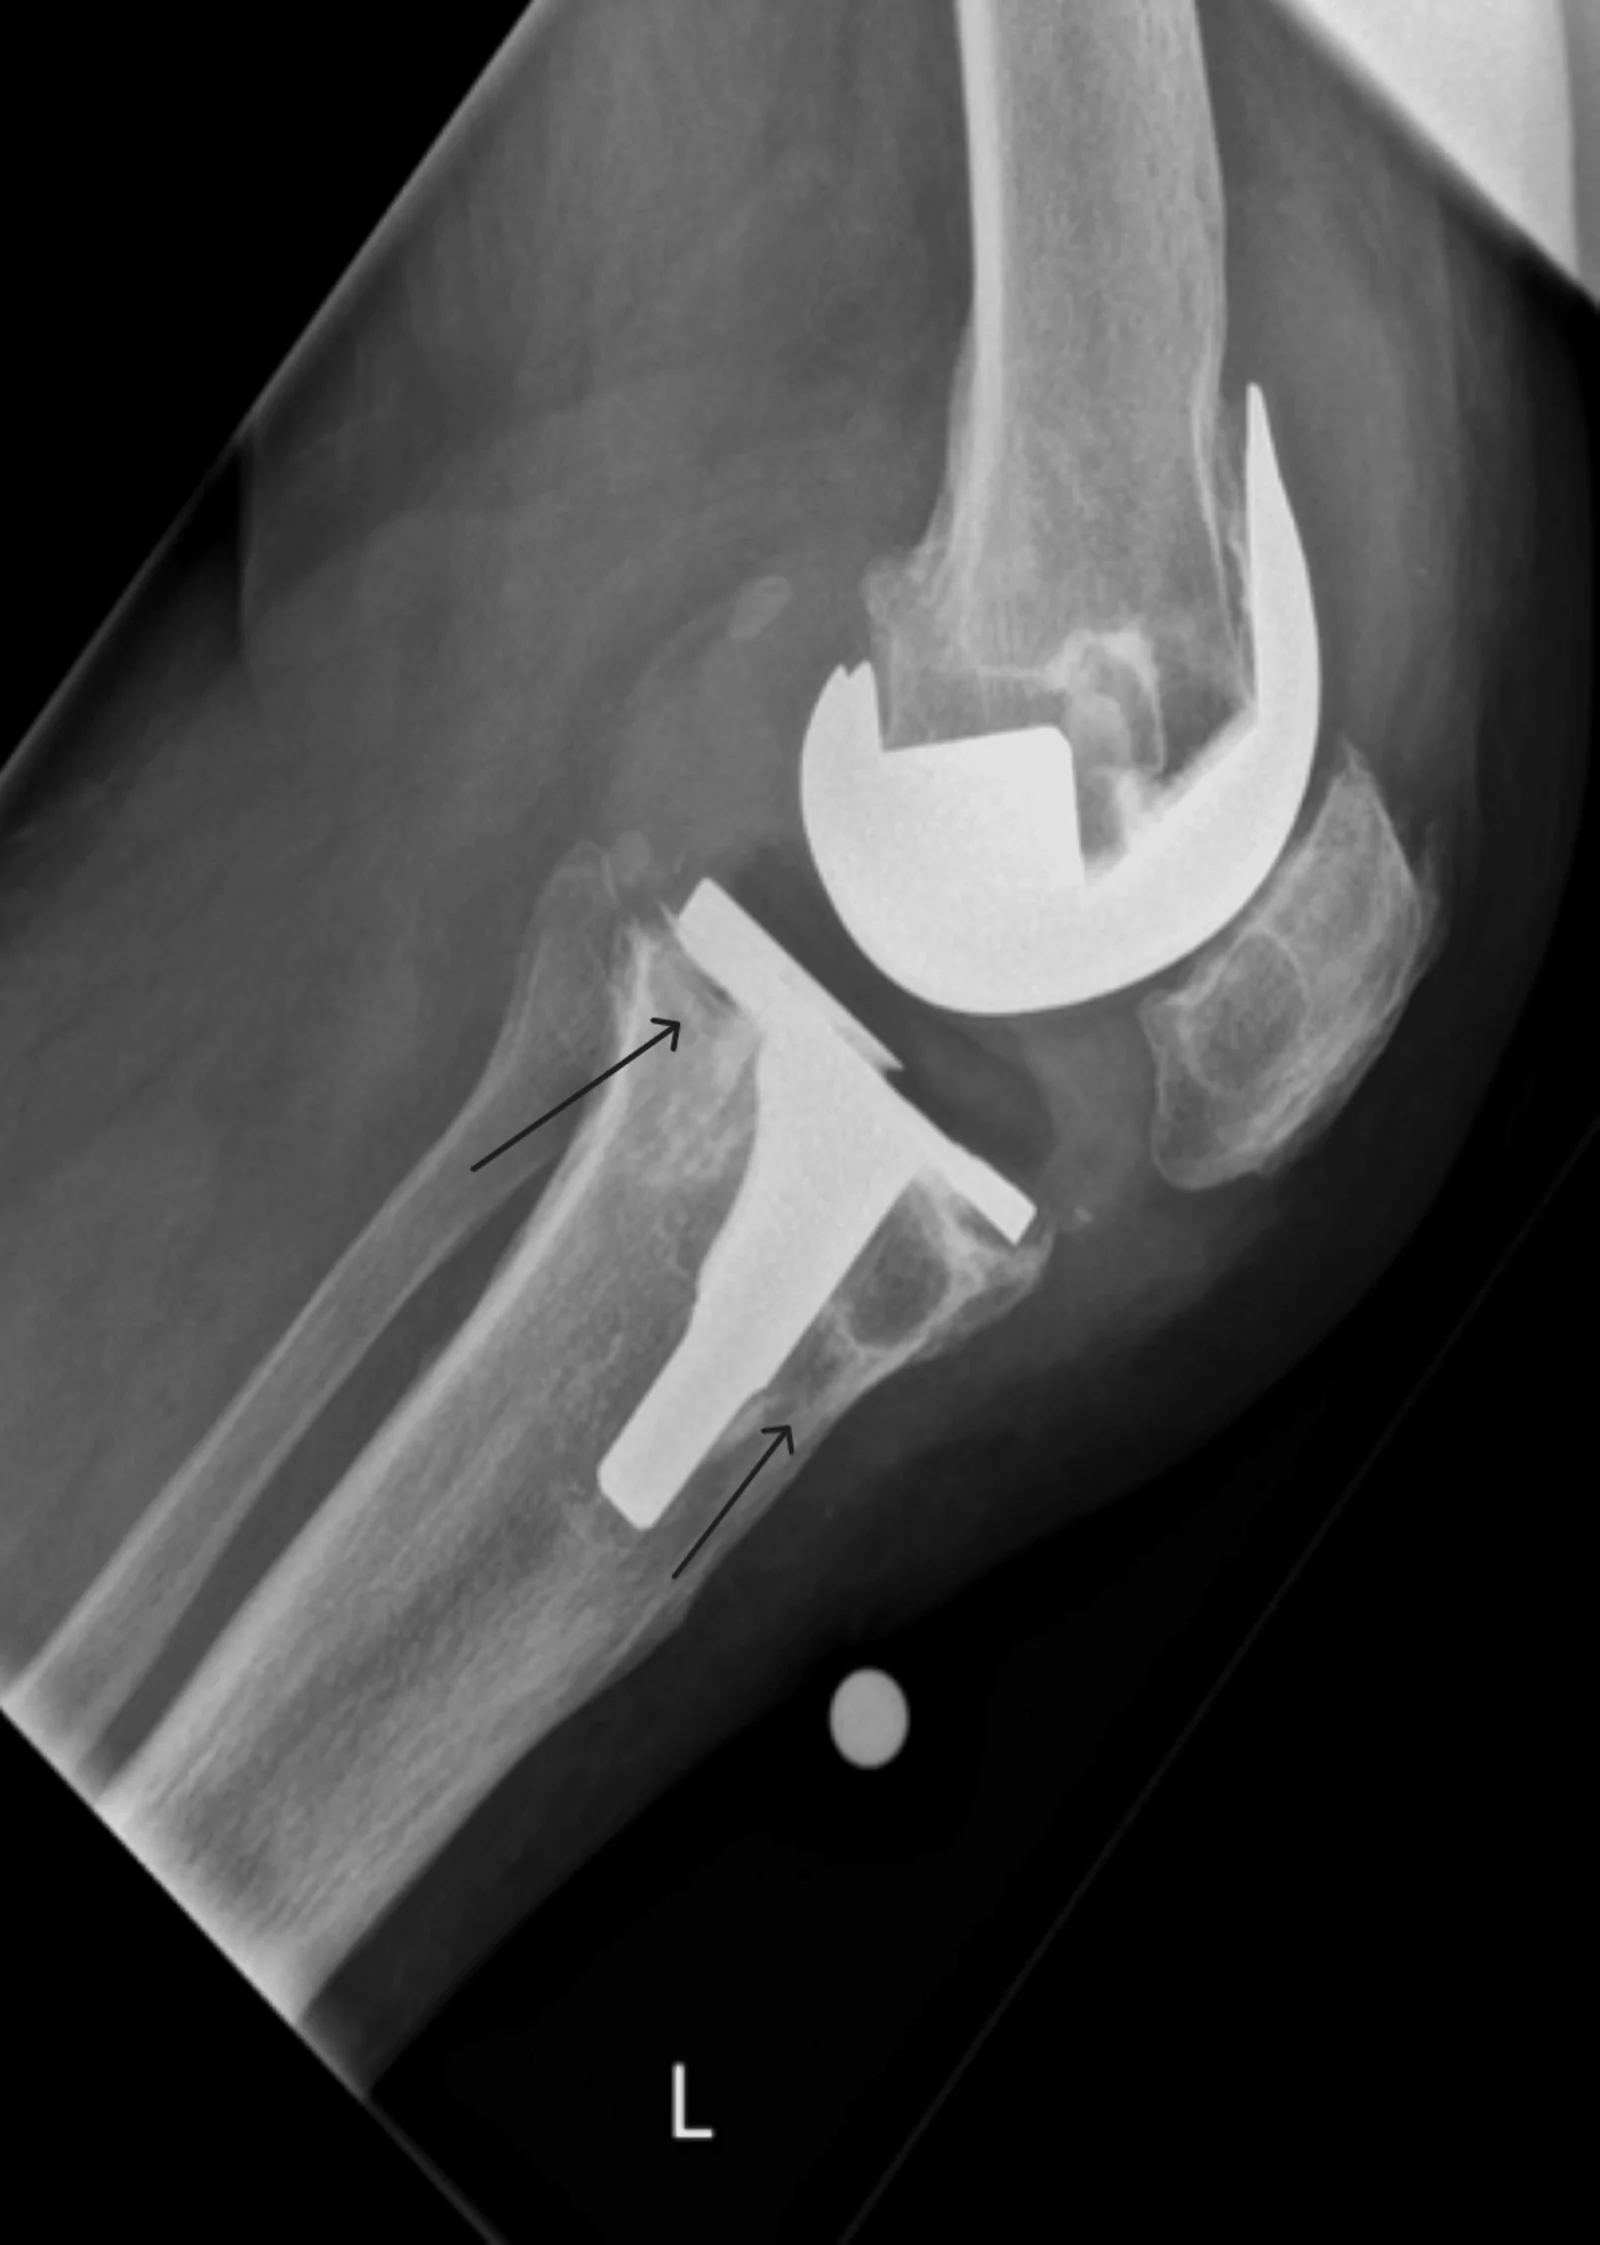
Lateral radiograph of the left knee showing minimal signs of osteolysis surrounding the TKA five years after primary TKA. TKA, total knee arthroplasty
